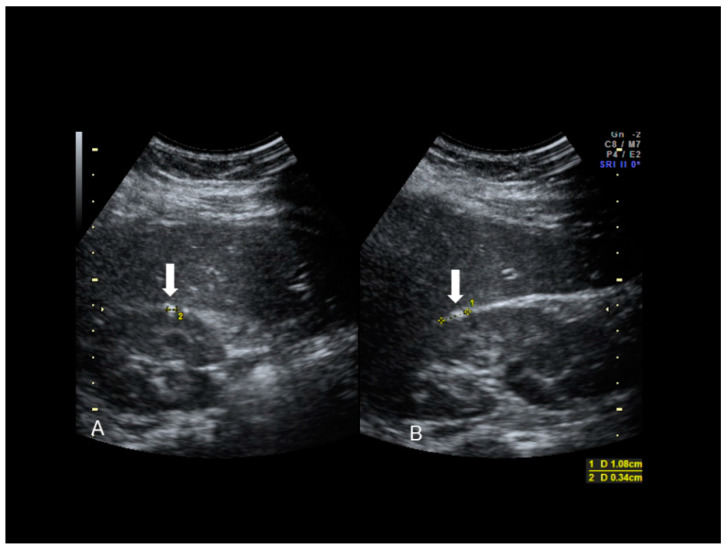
Endometriosis of kidney (see white arrow) confirmed at MRI (A,B).
